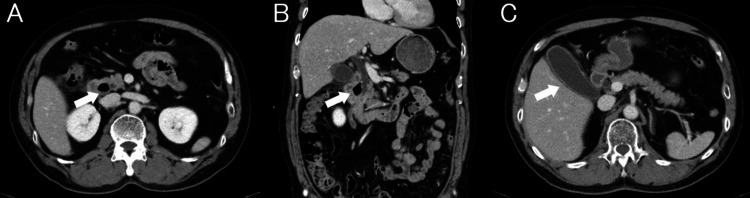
Contrast-enhanced abdominal CT performed during previous hospitalization for liver injury. (A) Axial and (B) coronal reformatted images demonstrate an air-filled periampullary duodenal diverticulum (white arrows) abutting the ampulla and compressing the distal common bile duct. The upstream extrahepatic bile duct is dilated. No choledocholithiasis is identified in the bile duct. (C) The axial image shows no gallstones within the gallbladder (white arrow).
